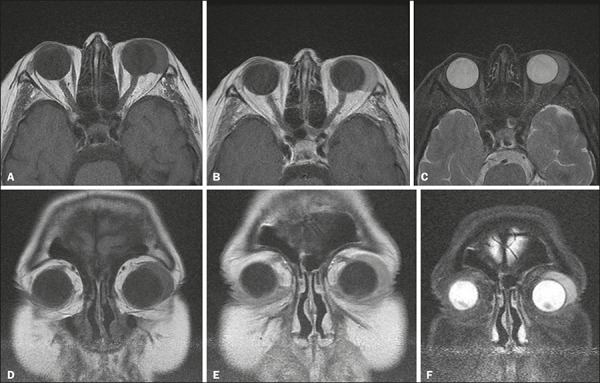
Axial and coronal MRI scans showing ocular lymphoma—axial T1W image
